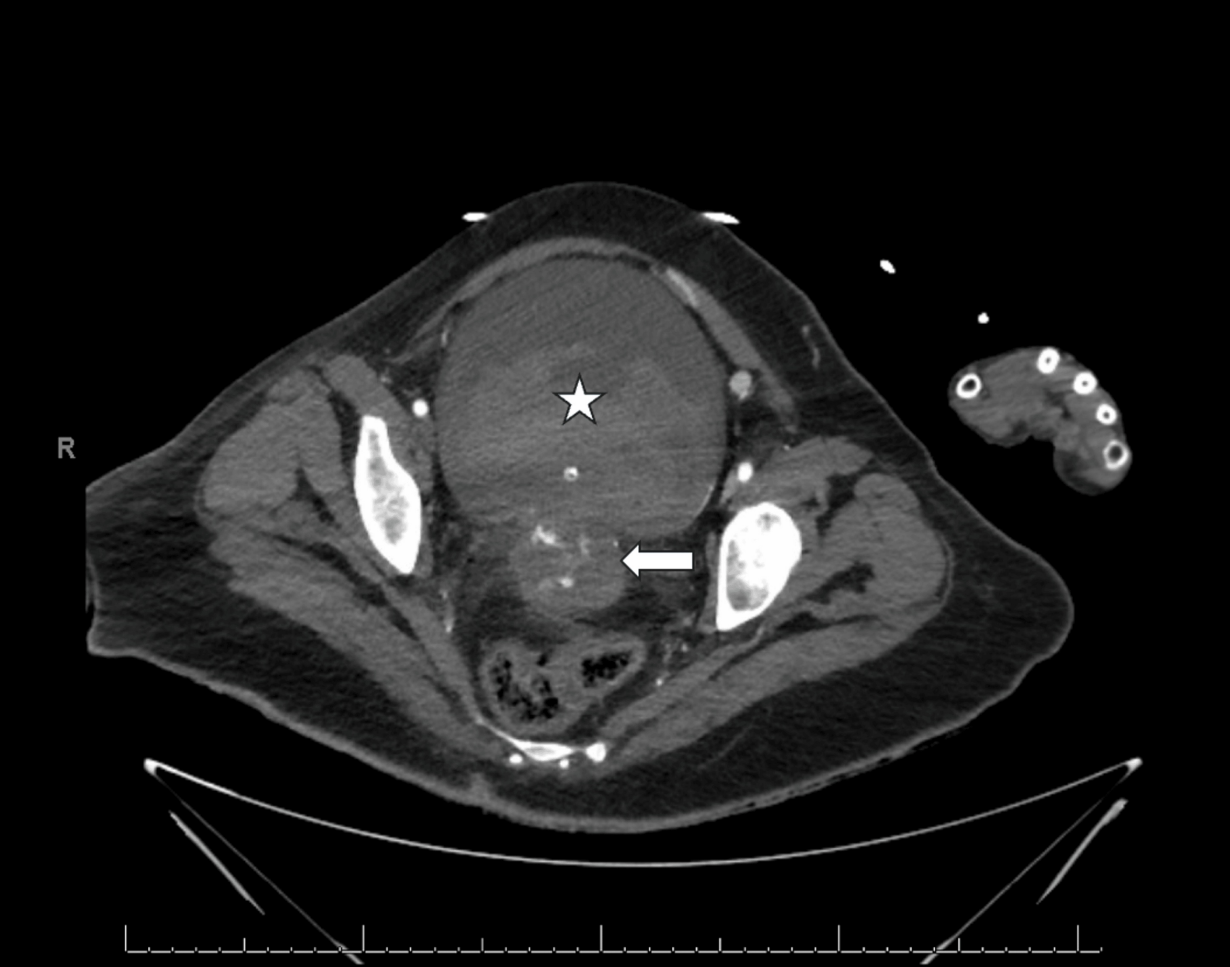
Large cervical mass with active extravasation (white arrow) and fistulous tract with bladder containing a large clot burden (white star) and Foley catheter on CT abdomen and pelvis with contrast axial view
